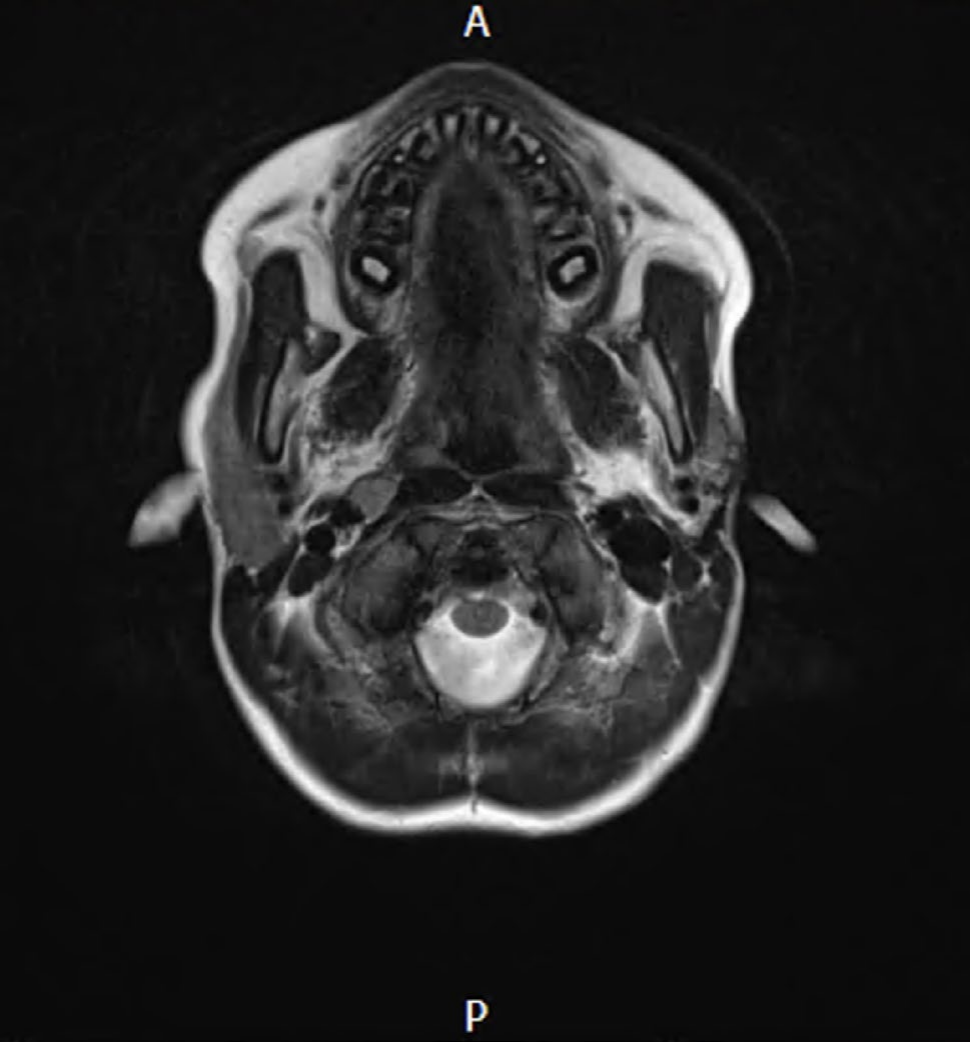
MRI imaging of post-operative recurrence on the leftside – axial view
